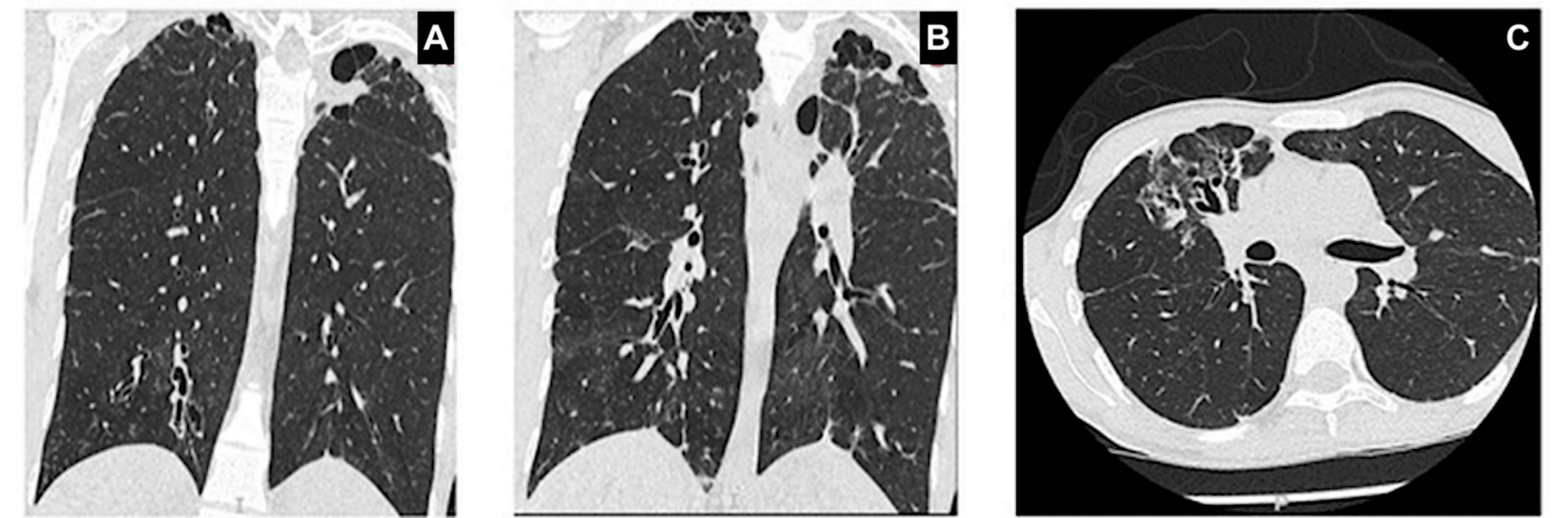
CT of the thorax demonstrating initial findings of structural lung disease (images represent the baseline CT findings, consistent with early bronchiectatic changes and inflammatory activity) (A) Coronal CT image showing left apical bullae formation; (B) coronal CT showing left apical bullae with evidence of cylindrical bronchiectasis, more pronounced in the right upper and middle lobes; (C) axial CT section demonstrating right-sided cylindrical bronchiectasis associated with ground-glass opacities, suggestive of active inflammation or infection.
